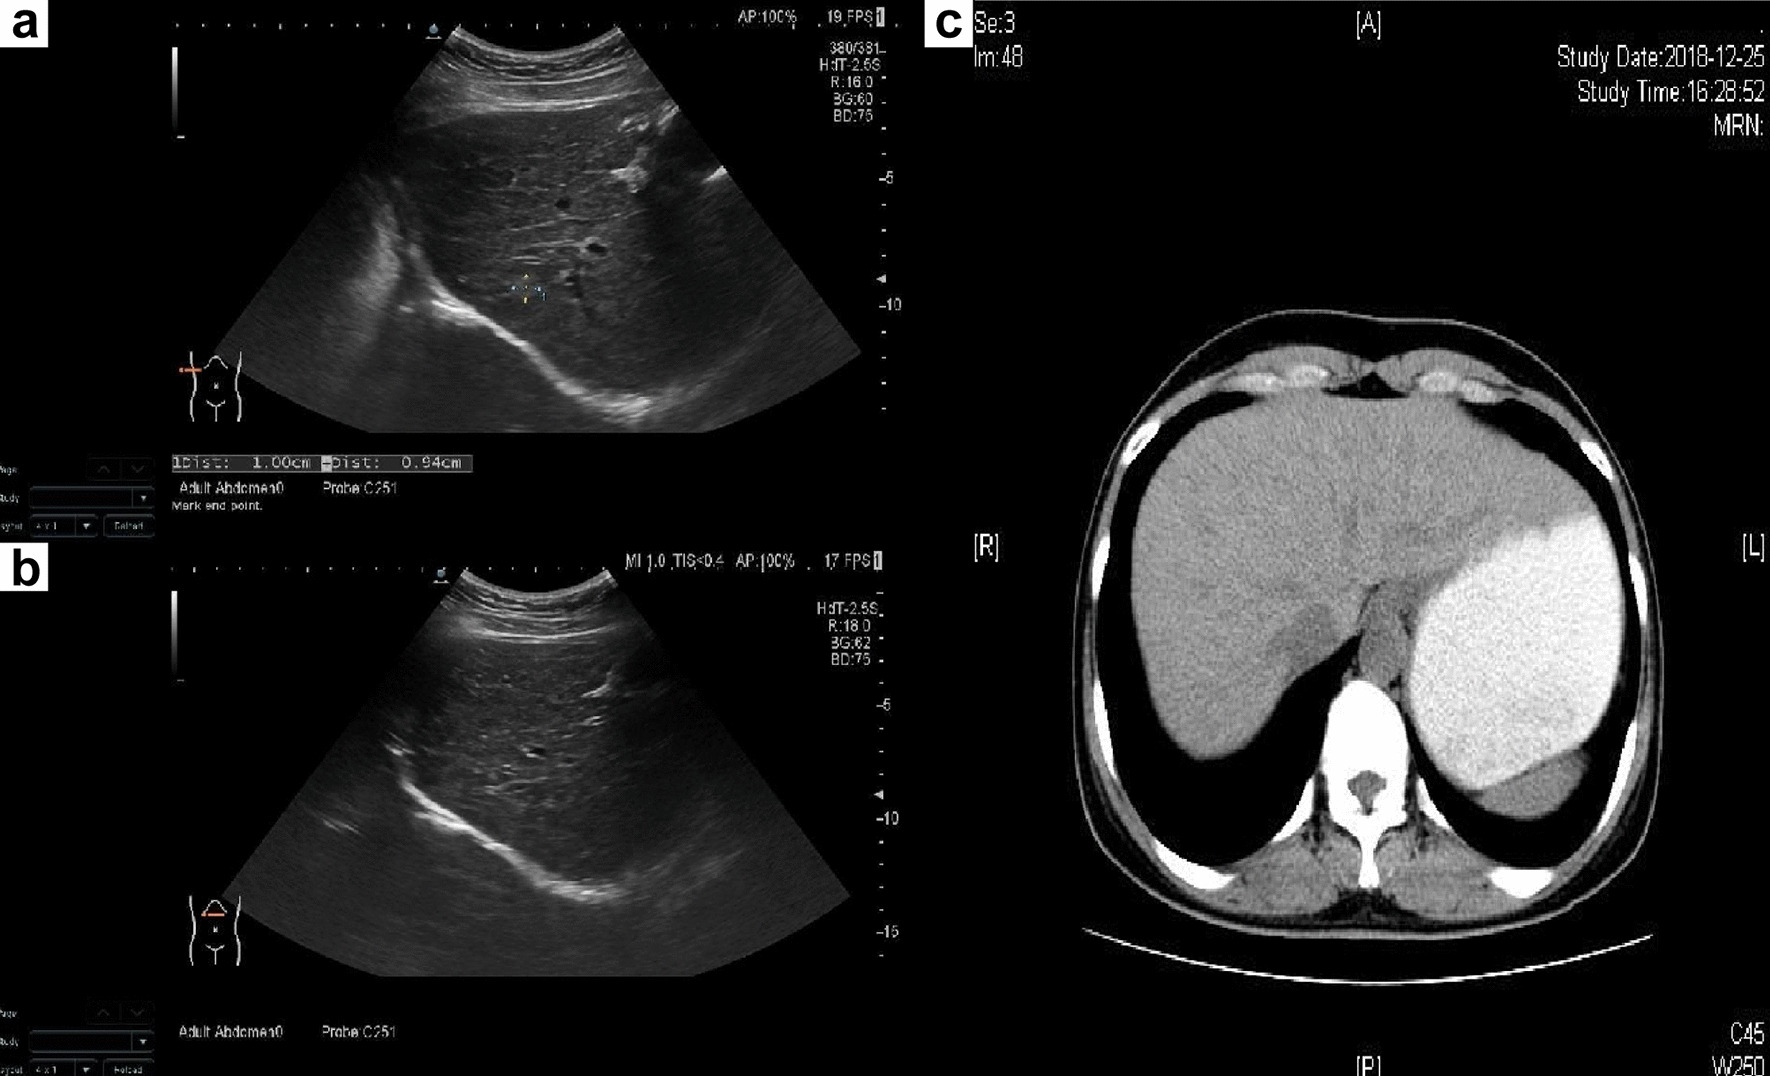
Abodominal ultrasound and CT images of the patient showing early liver cirrhosis. a, b Abodominal ultrasound revealed unsmooth liver surface, irregular edges, enhanced thickening dot echoes and uneven distribution with several slightly hyperechoic nodules. c CT of the upper abdomen showed that the hepatic capsule was wavy
